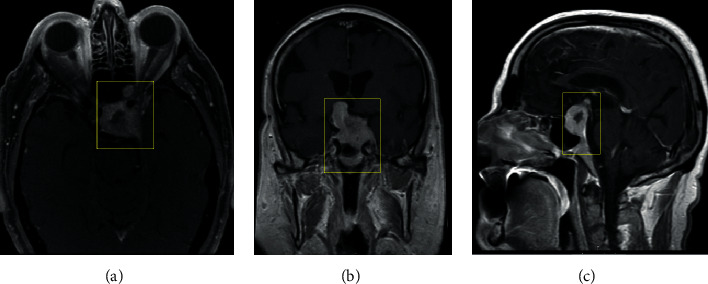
Radiographic imaging, on initial workup of the heterogeneously enhancing eccentric. T1-weighted sequence magnetic resonance imaging with contrast presented in axial, coronal, and sagittal planes, respectively. The mass invades the left cavernous sinus and orbit with envelopment of the left internal carotid artery (a). In the suprasellar region, the mass extends into the right hypothalamus (b) with mass effect on the third ventricle (c). The mass measures 4.3 x 3.3 x 3.7 cm with a calculated volume of 17.11 mL using 3D modelling. Boxes highlight the tumor of interest.
